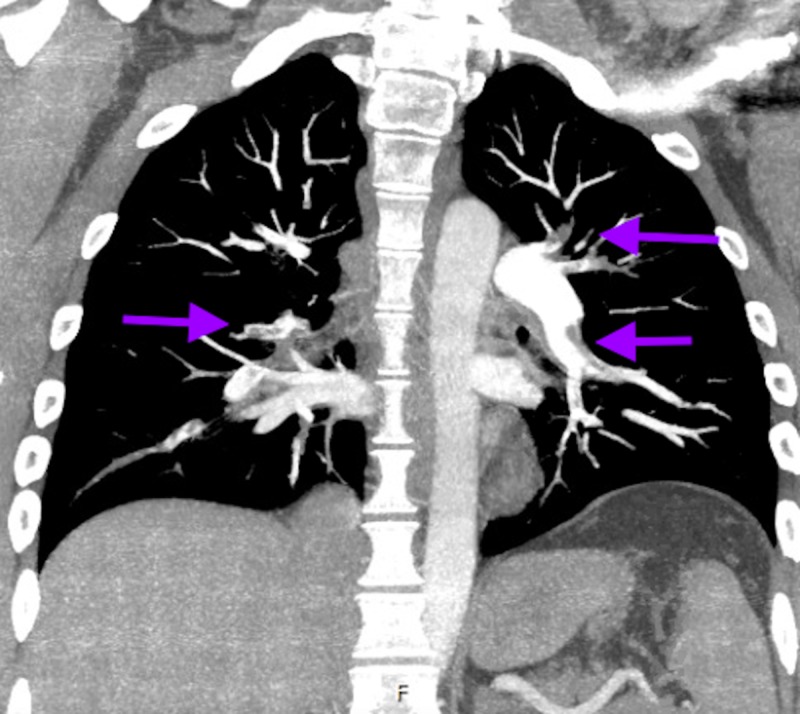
Computed tomography angiography (CTA) of the chest showing bilateral segmental embolism
